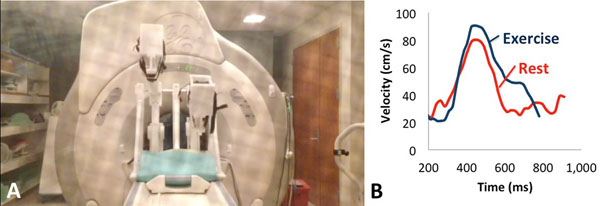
(A) MRI-exercise device with volunteer exercising in scanner. (B) Mitral valve flow-time curves at rest and during exercise in a healthy subject. The E/A ratios decreased and the E-wave deceleration times increased with exercise.
